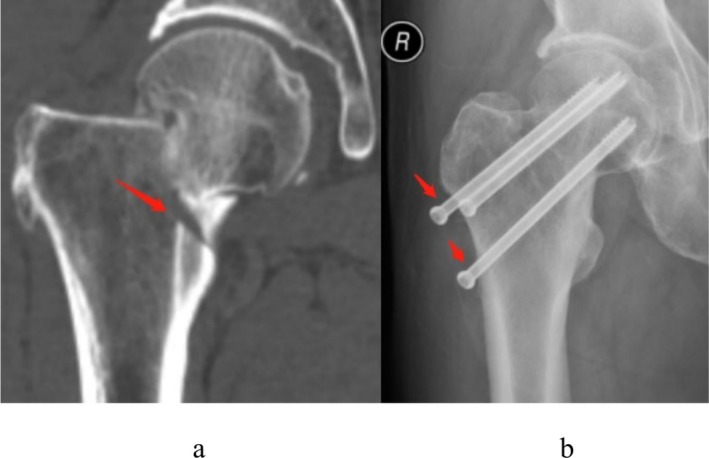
Radiographic findings in a femoral neck fracture case: (a) initial imaging demonstrating calcar femorale injury (red arrow); (b) subsequent screw migration due to femoral neck shortening (red arrows).
